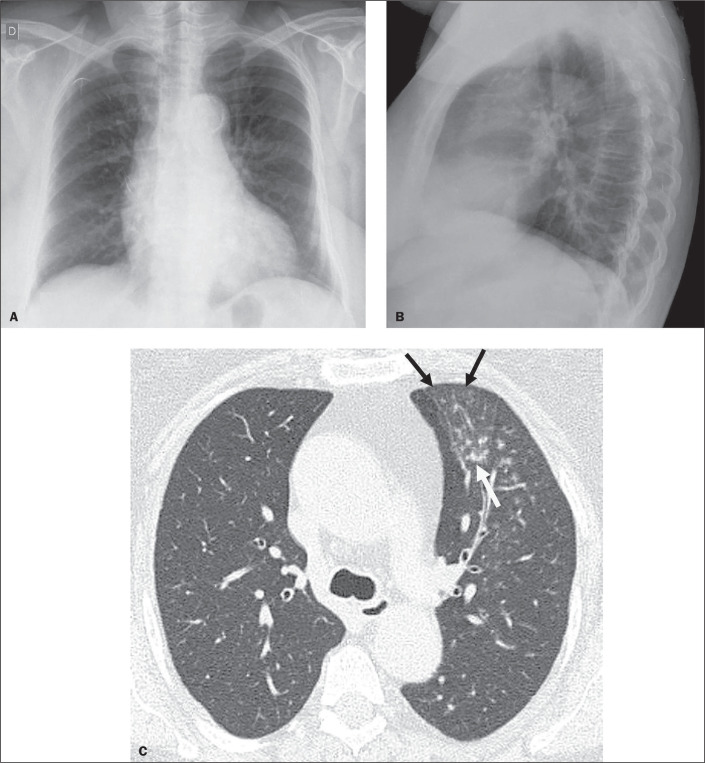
Chest X-ray (A,B) and HRCT (C) of a 53-year-old female with pulmonary
